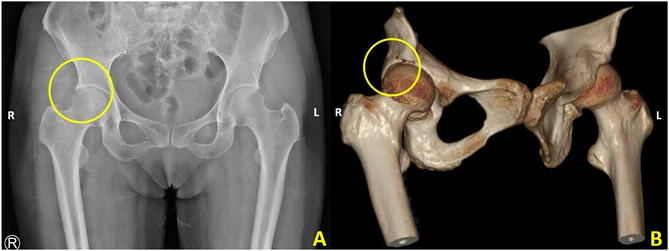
Postoperative imaging examinations. (A) Weight‐bearing pelvic anteroposterior radiograph, showing elimination of the right hip impingement structure; (B) 3‐dimensional computed tomography reconstruction of the hip, showing good anchor position in the right hip, consistent with the intraoperative plan.
